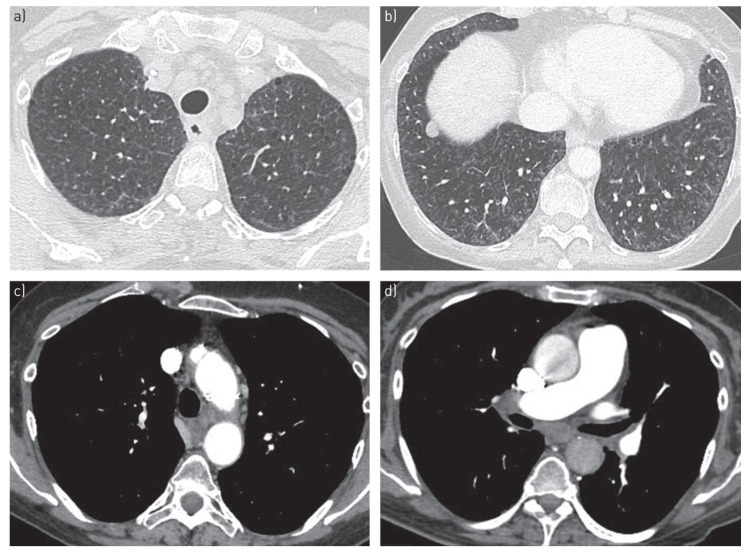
HRCT of patient with PVOD. Images (a,b) display centrilobular ground-glass opacities. Images (c,d) display mediastinal lymphadenopathy.
